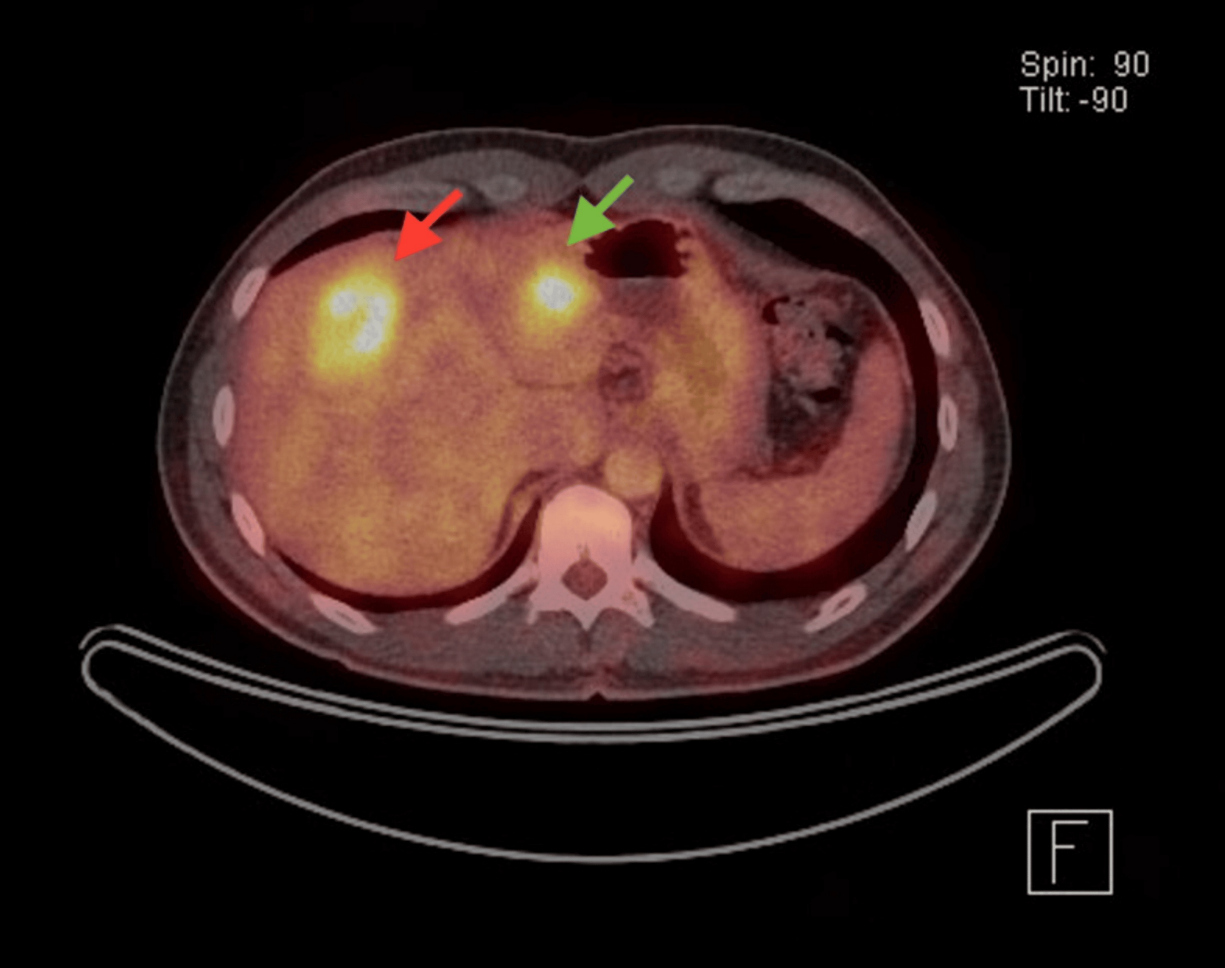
Initial PET-CT axial plane Red arrow: Lesion in segment 8-4a. Green arrow: Lesion in segment 2. PET-CT: positron emission tomography-computed tomography
